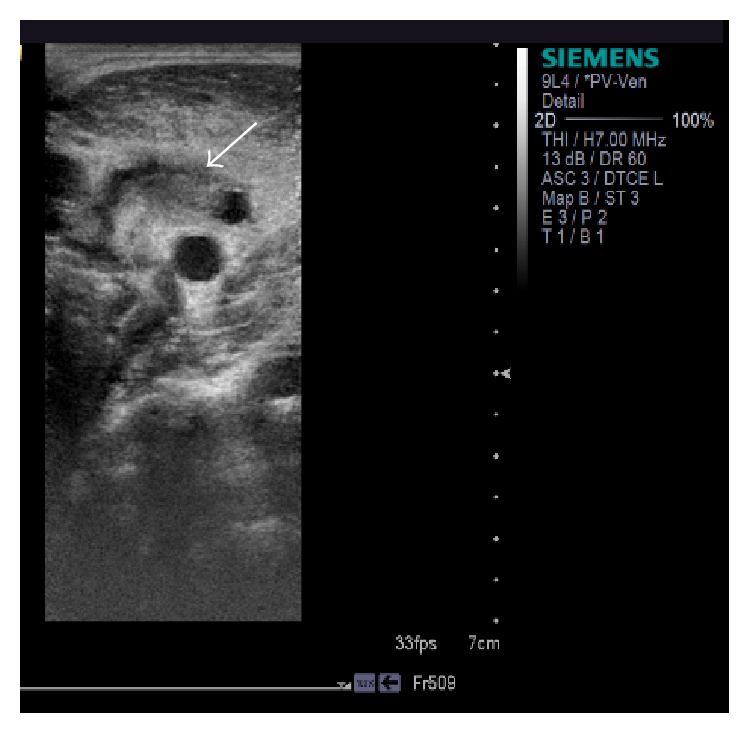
Neck ultrasound: intraluminal thrombus of the IJV (white arrow) with inability to fully compress the vein.
